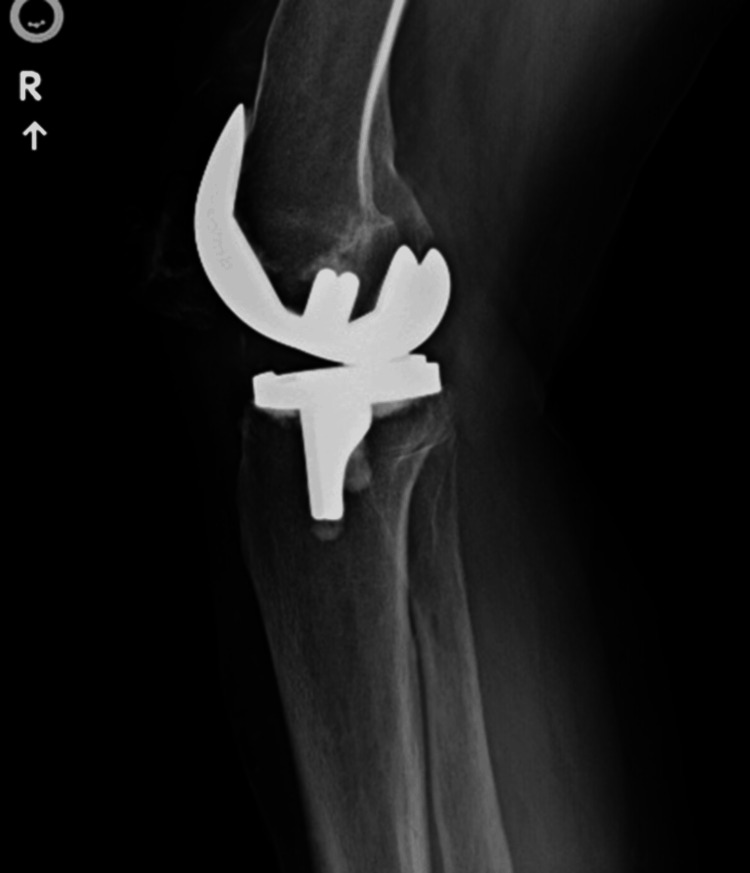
Preoperative lateral radiograph of the right knee demonstrating resorption of the right patella
